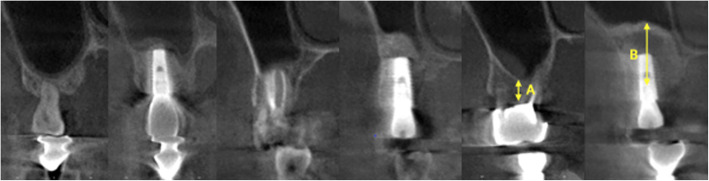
Cone-beam computerized tomography (CBCT) of patient shown in Figs. 2 and 3. A Residual bone height. B Increased bone height
